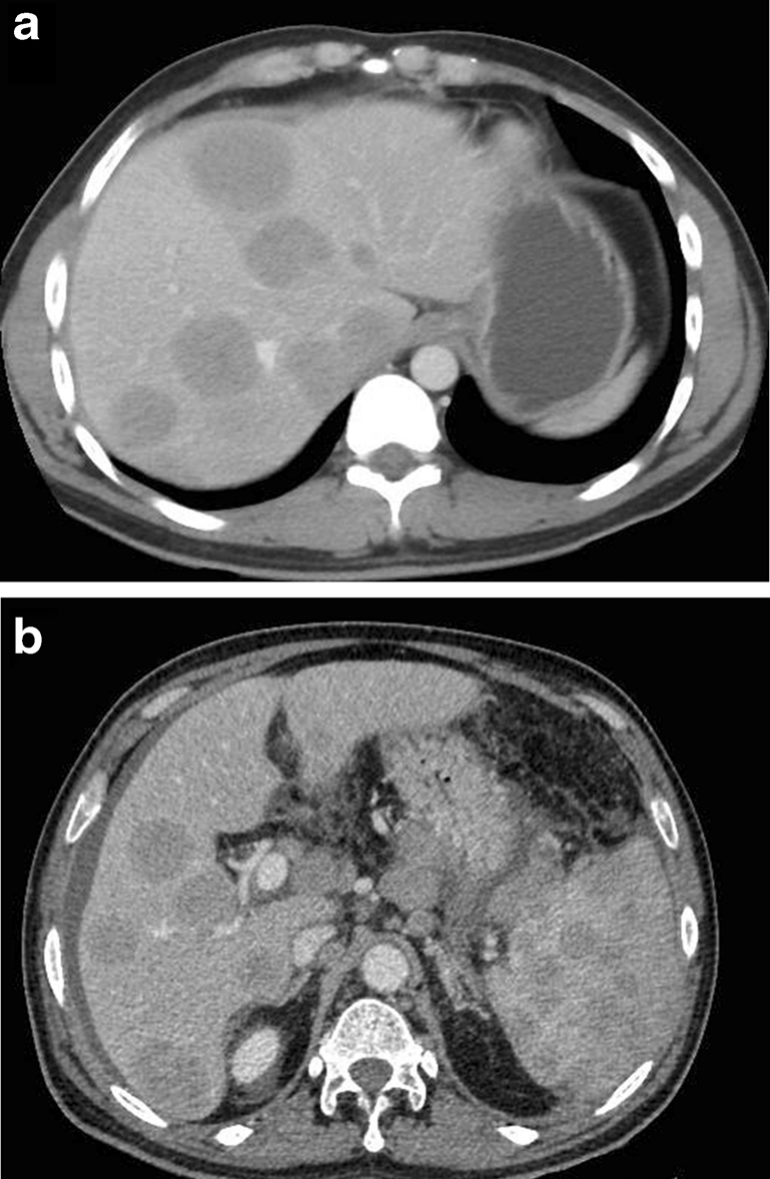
CT findings in SHL from two different patients. Axial CECT images demonstrating multiple homogeneous hypoenhancing hepatic lesions. Splenic lesions and perihepatic lymph nodes can also be seen in the image b
